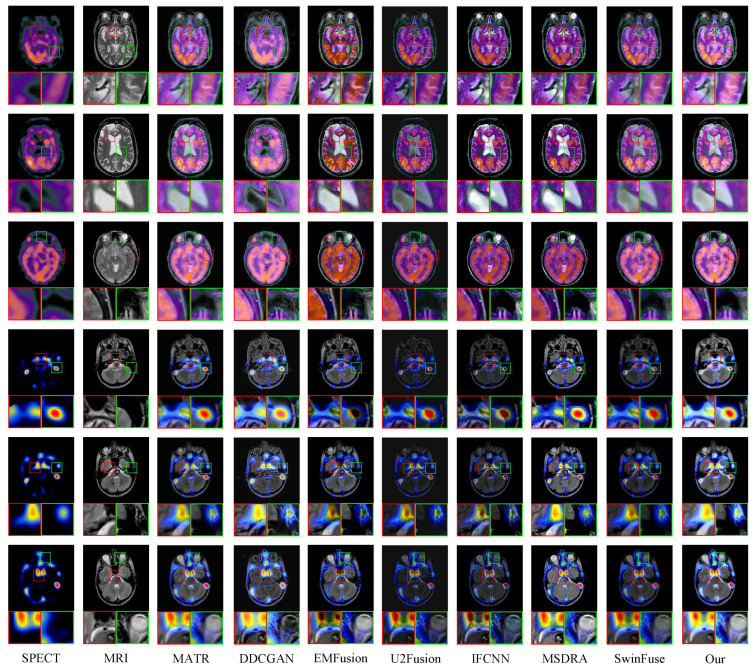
Qualitative evaluation of the proposed MDC-RHT and seven typical and state-of-the-art methods on six representative SPECT and MRI image pairs. From left to right: SPECT, MRI, MATR, DDCGAN, EMFusion, U2Fusion, IFCNN, MSDRA, SwinFuse, and MDC-RHT.
